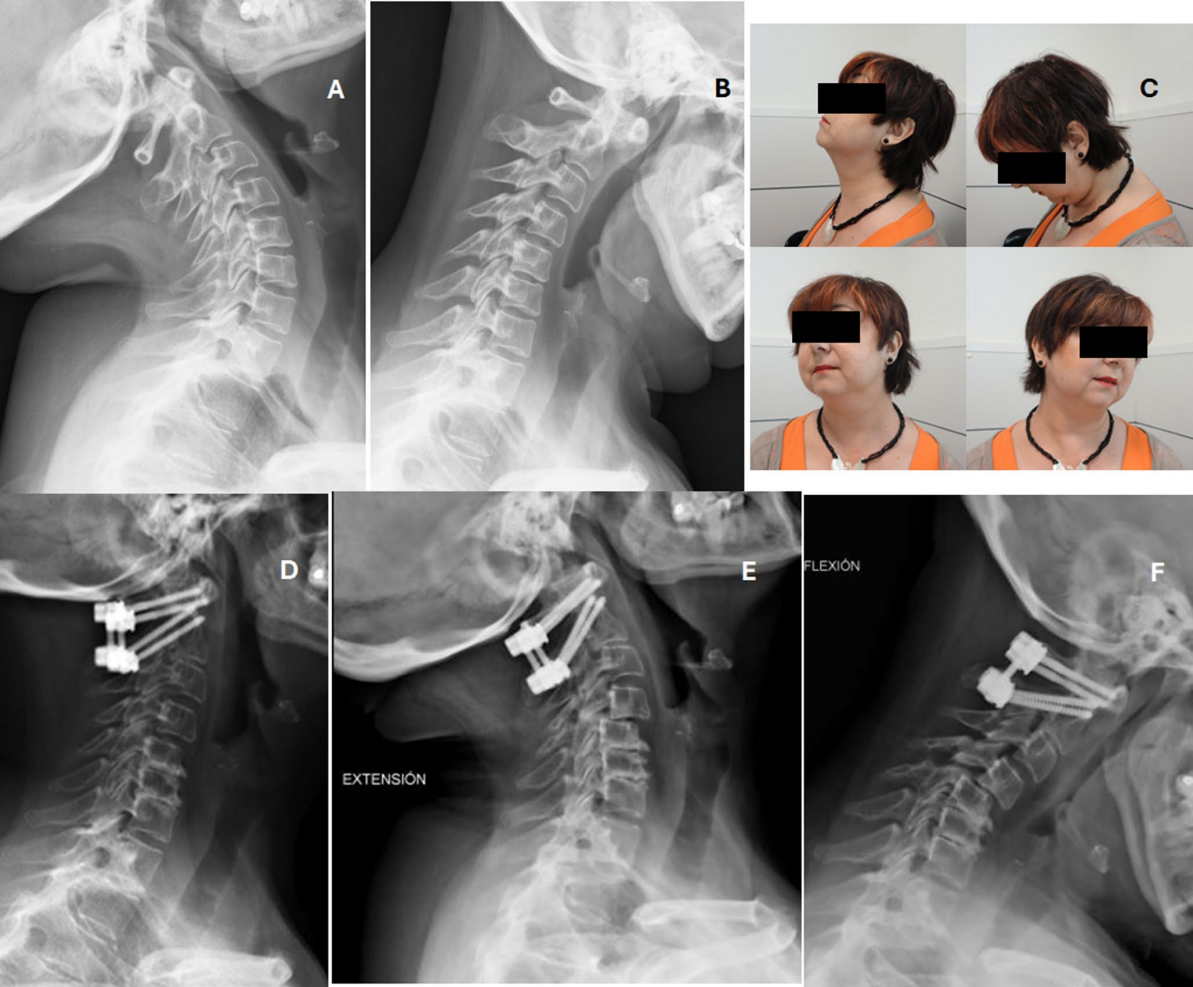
Case 10, Table 3. 50-year-old female with reducible Atlanto-Axial subluxation without neurological affectation. A and B: dynamic flexion-extension shows Atlanto-Axial instability. There was no subaxial involvement at the year 2010; C: Clinical results at six months post-operation; D: Five-year follow-up lateral XR shows C5-6-7 affectation and a slight subluxation C4-5 in this static radiograph; E and F: In January 2025, fifteen years after the index operation, an instability C4-C5 due to the progression of the disease is clearly seen in dynamic radiographs
